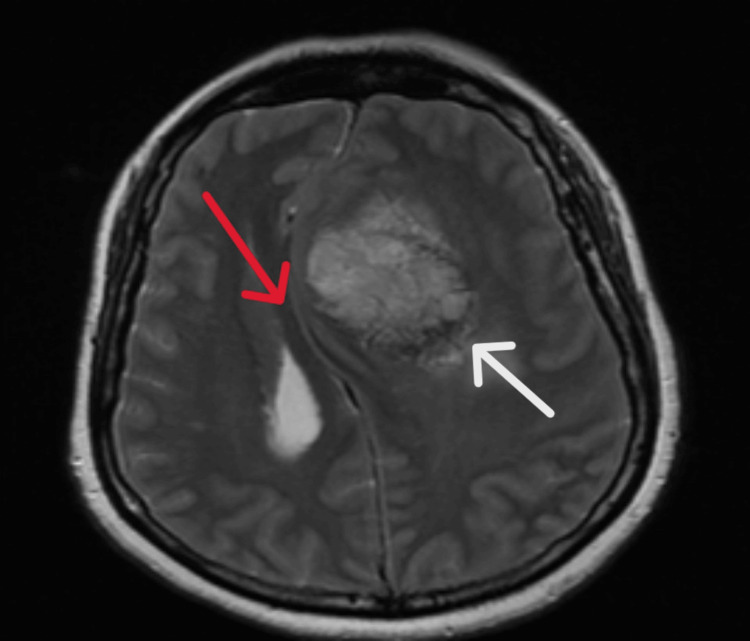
A brain MRI demonstrating a left frontal mass (white arrow), with surrounding oedema and mass effect on the midline dura (red arrow). MRI, Magnetic Resonance Imaging
